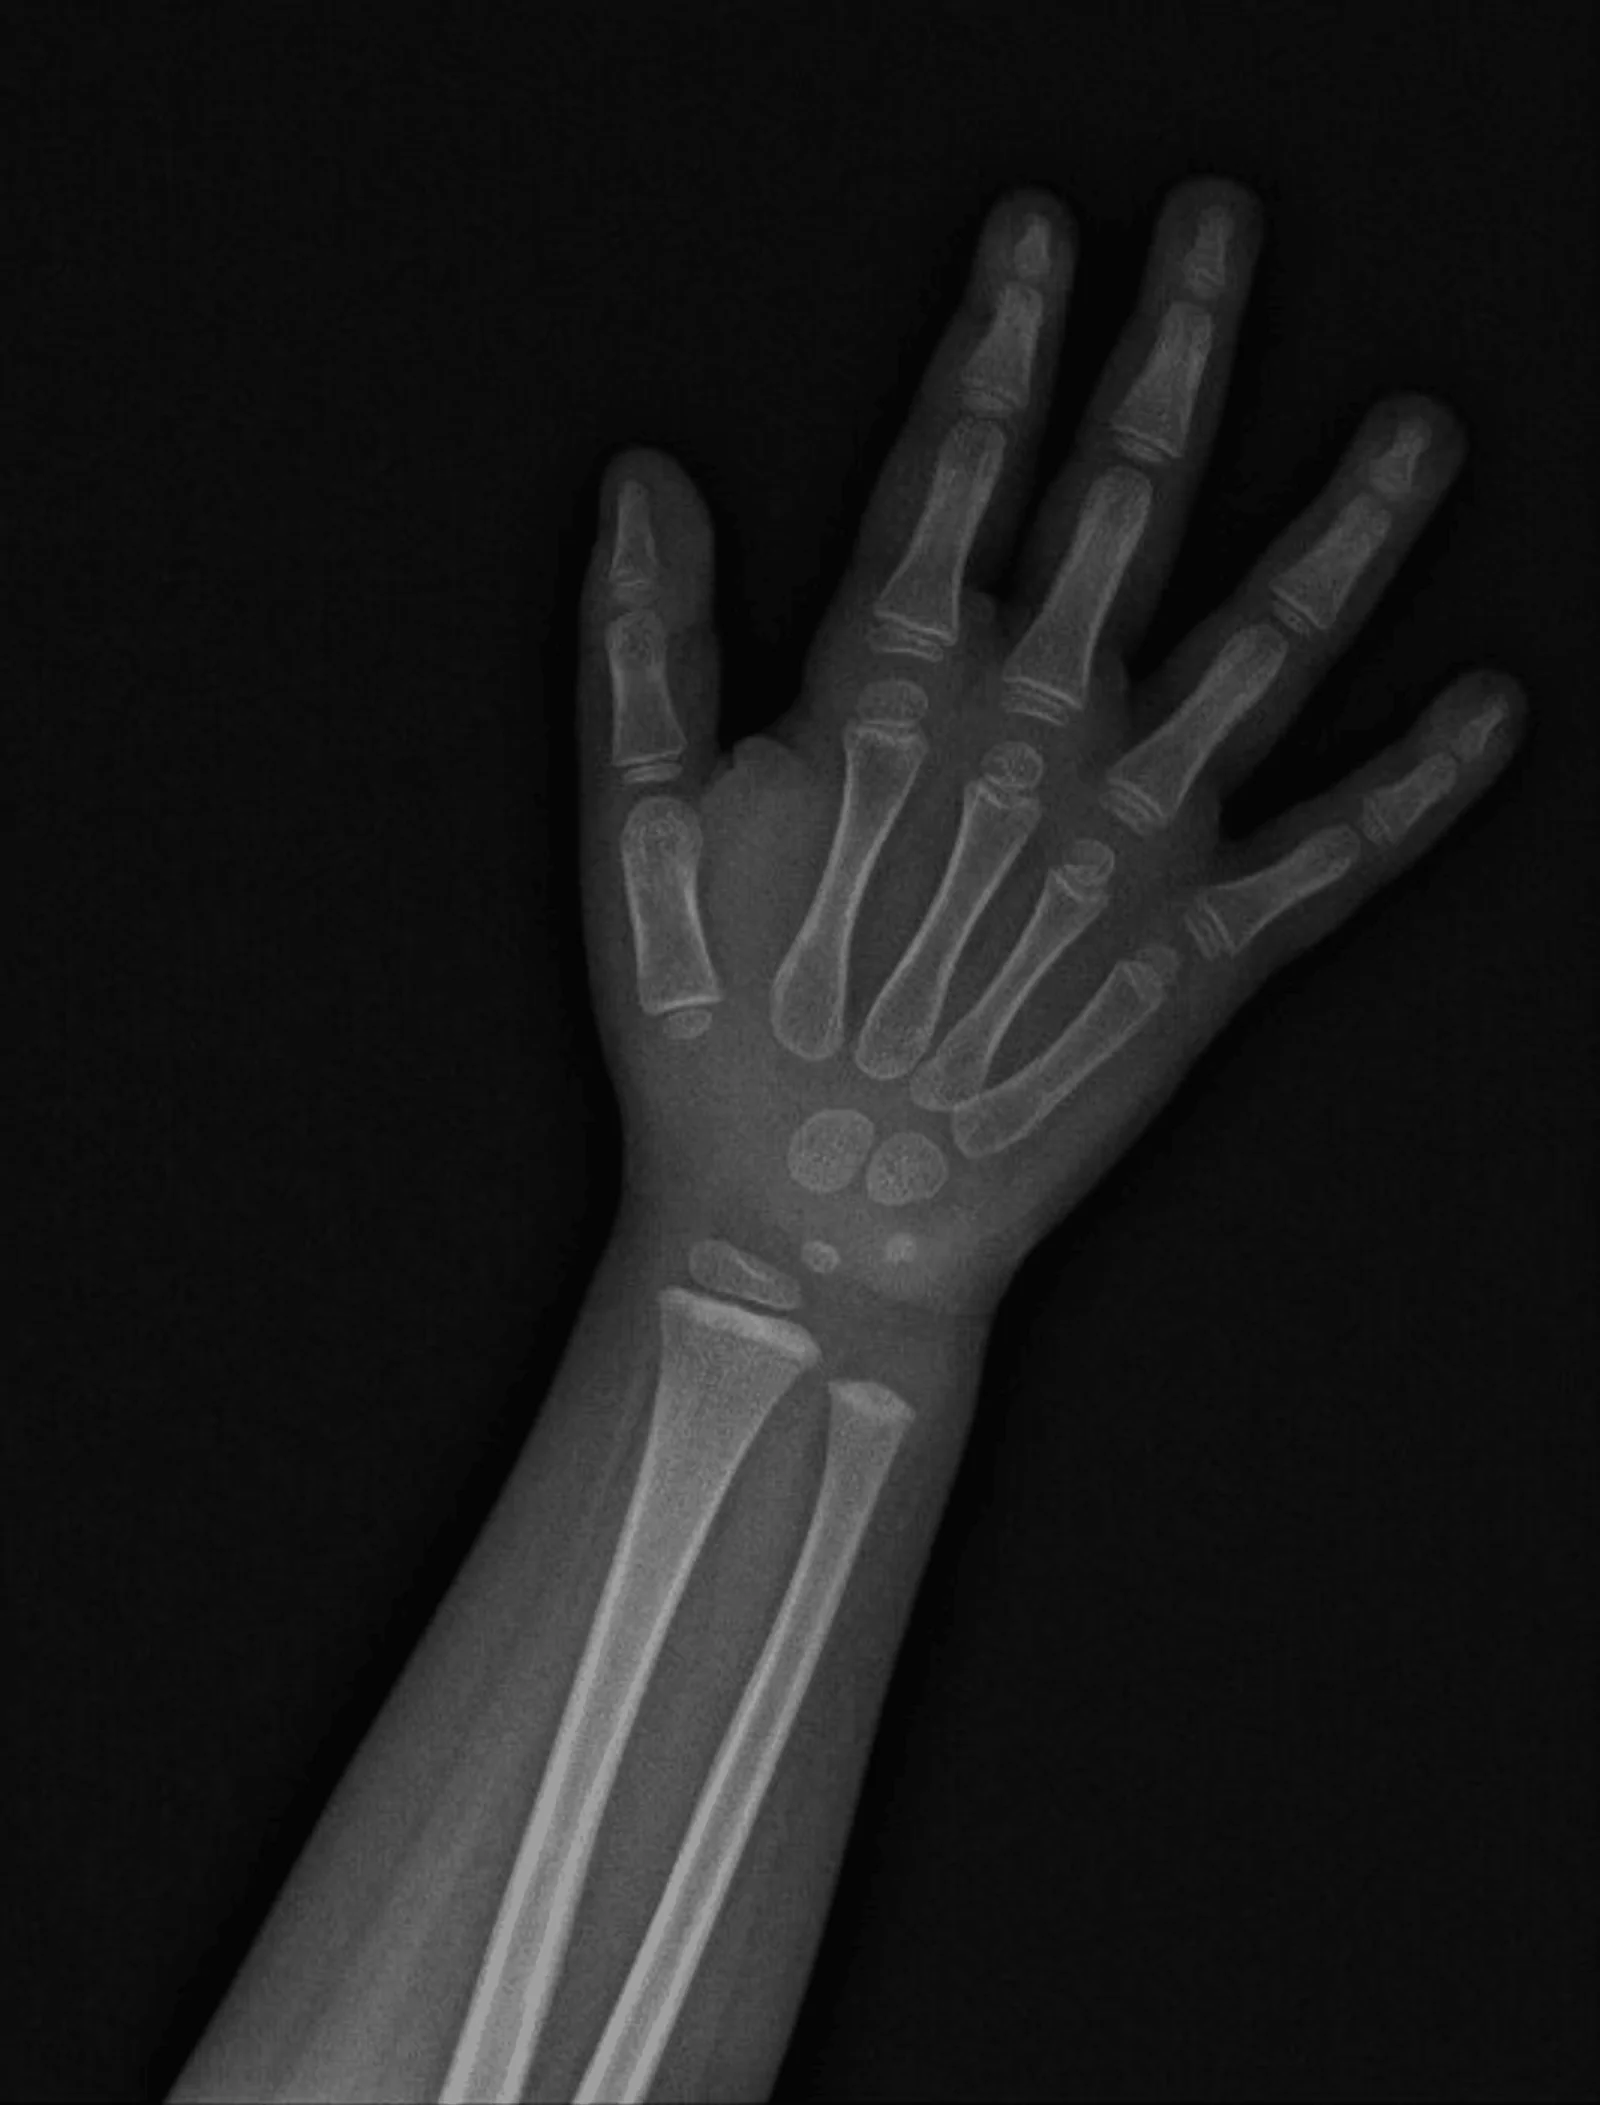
Anteroposterior Radiograph of the Right Hand and Wrist No acute fracture is identified, and osseous alignment is preserved.
